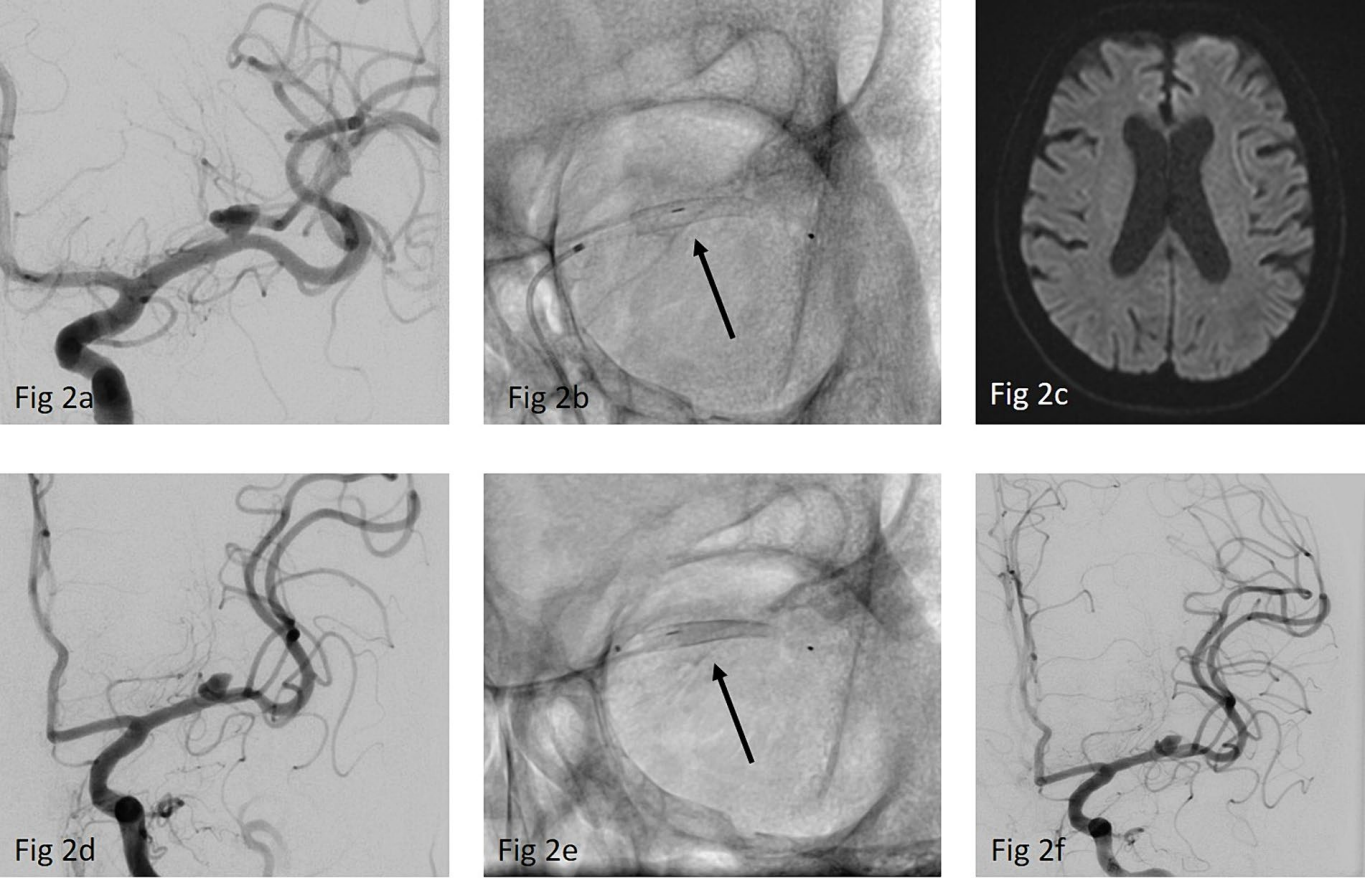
(a) ap-view of a left sided MCA-bifurcation aneurysm with a dominant inferior trunk (b) implantation of a p48 MW HPC 3/12 mm (arrow) over a Rapid Transit microcatheter (c) MRI prior to discharge showed no DWI lesions (d) DSA- FU after 12 months showed only a slight reduction of the aneurysm size while the caliber of the jailed superior trunk also remained nearly unchanged (e) Re-treatment of the aneurysm by implanting a second p48 MW HPC 2/15 mm (arrow) (f) final run after implantation of the second FDS showing no delayed perfusion of the jailed branch. FU is not yet available.
